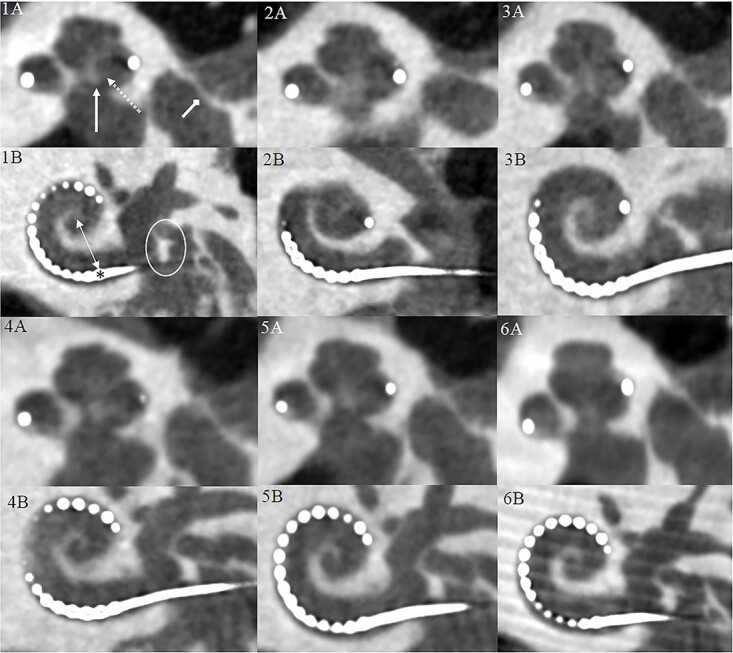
The inner ear structures evaluated from the CBCT images. Two views of each imaging protocol, which is indicated by the number in the upper left corner of each figure, are presented. The osseus spiral lamina (dashed arrow), the modiolus (solid arrow) and stapes footplate (diamond-headed arrow) can be evaluated from the view ‘A’. The most basal electrode (asterisk) whose distance from the round window and the modiolar axis (two-headed arrow) as well as the round window niche (encircled) can be assessed from the view ‘B’. The cochlea and the modiolus (A, solid arrow) can be evaluated from both views.
